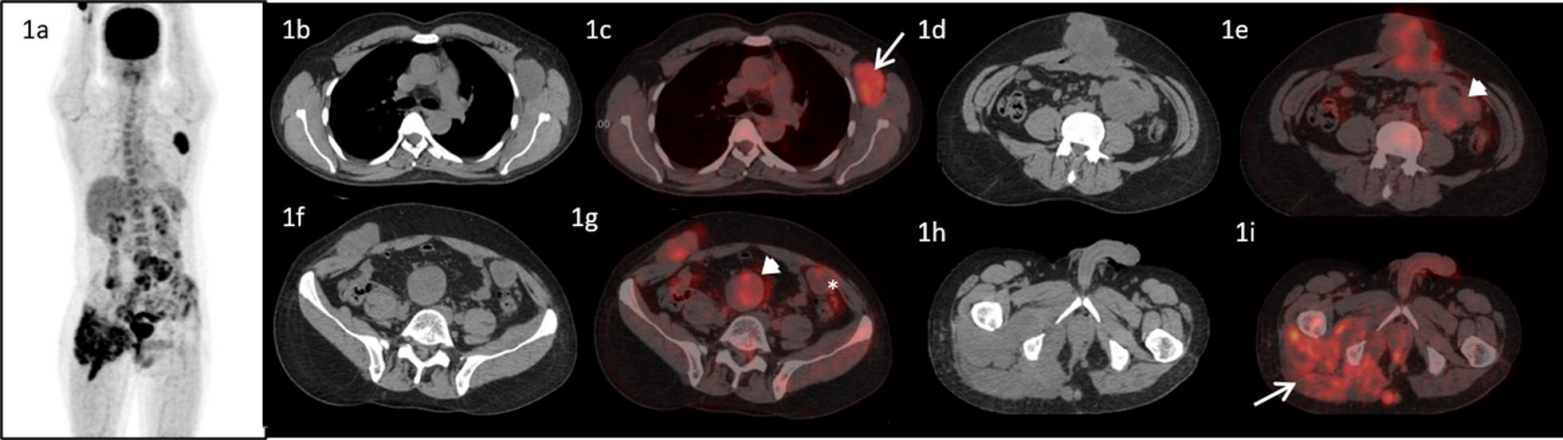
A pre-imatinib 18F-Flurodeoxy glucose (FDG) positron emission tomography/computed tomography (PET/CT) maximum intensity projection (MIP) image of the patient showing increased FDG uptake in the left axillary, lower abdominal and pelvic regions. Transaxial computed tomographic images at the level of axilla, L3 vertebra, L5 vertebra and ischial tuberosity (1b, 1d, 1f and 1h) and corresponding fused PET/CT images (1c, 1e, 1g and 1i) showing enlarged and FDG avid left axillary lymph node (1c, arrow), FDG avid lobulated mass in the anterior abdominal wall (1d and 1e), deposit in the right external oblique muscle (1f and Ig), multiple mesenteric (arrow heads) and omental* deposits (1e and 1g). There was soft tissue thickening adjacent to the right ischium involving the right pyriformis and gluteal muscles with increased FDG uptake and soft tissue deposit the subcutaneous plane adjacent to it (1h, 1i -arrow)
